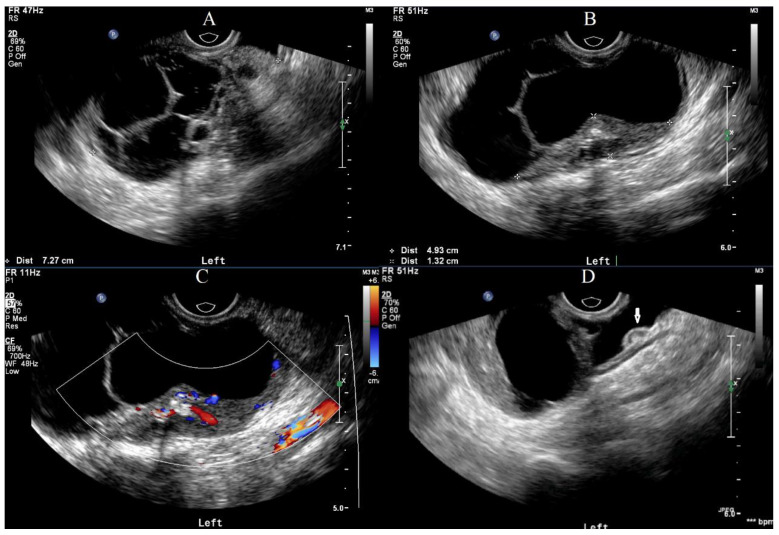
Ultrasonic features of the mass. (A) A multilocular solid-cystic mass with a clear boundary was seen in the left adnexal area, in which lipid and hair-like hyper-echo mass was also observed. (B) Calcification can be seen in solid hypo-echo of the mass. (C) The CDFI revealed scattered, short strips of blood flow signals in the solid proportion of this mass. (D) Implant nodules can be seen on the intestinal surface.
